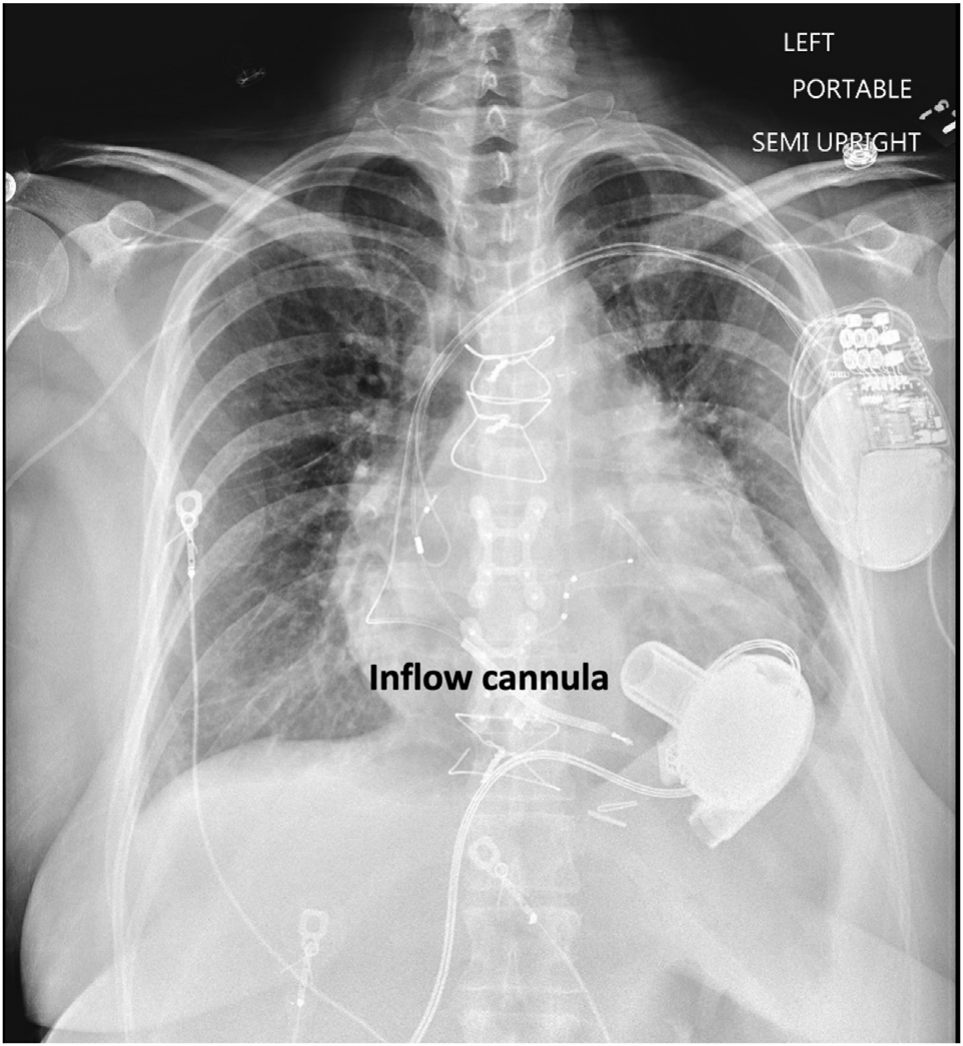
Portable, semiupright posterior-anterior chest radiograph at admission demonstrates mild, diffuse bilateral pulmonary edema and stable, unchanged position of the LVAD inflow cannula compared with prior; also shown is the left-sided cardiac resynchronization therapy device with defibrillator and right arm peripherally inserted central catheter.
